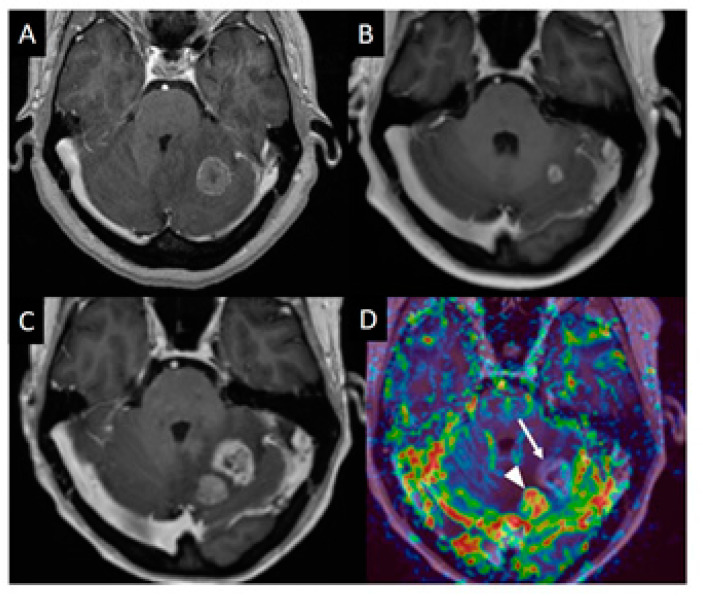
T1-weighted and DSC imaging of a left cerebellar metastasis. (A) Post contrast T1-Weighted MRI image of a 40 yo (year old) female with colon cancer and left cerebellar metastasis. (B) MRI 7 months later showed marked reduction is size after radiosurgery treatment. (C) MRI performed 11 months after treatment showed increase in size of the treated lesion and a new enhancing lesion medial to it. (D) CBV map from DSC perfusion (arrow) demonstrates mainly low perfusion. Some small foci of slightly elevated perfusion may represent foci of recurrent tumor on a background of predominant radiation necrosis.
